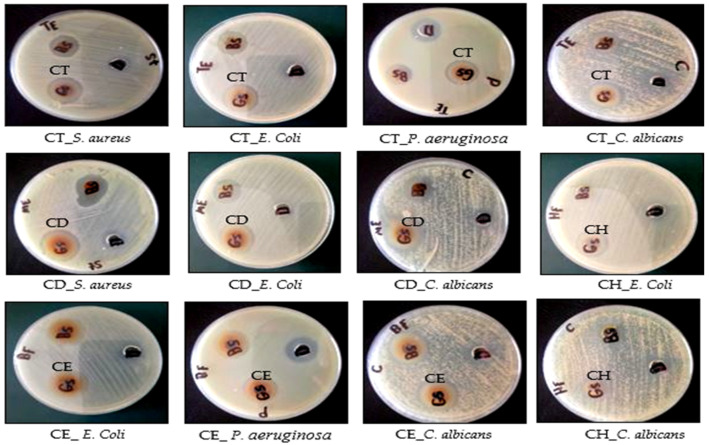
Antimicrobial activity of *Cliona* sp. Total extract (CT), *n*-Hexane (CH), Dichloromethane (CD), and Ethyl acetate (CE) fractions by agar dilution method with *S. aureus*, *E. coli*, *P. aeruginosa*, and *C. albicans*. Ciprofloxacin (antibacterial) and Fluconazole (antifungal) were used as positive controls while DMSO was the negative control.
